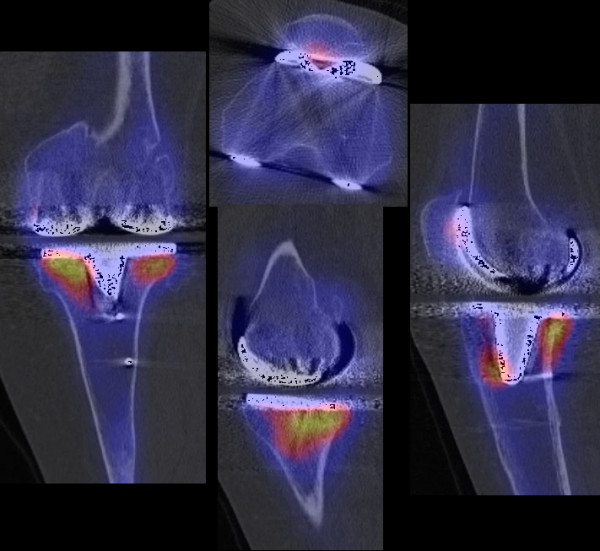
SPECT/CT imaging of a patient with mechanical loosening of tibial component as cause of painful total knee arthroplasty.
